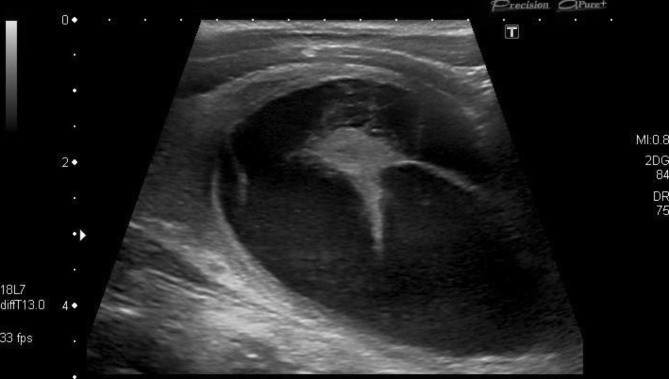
Ultrasound of the thyroid gland Ultrasound prior to sclerotherapy, one year earlier. Note the large bleeding cyst in the right thyroid lobe
